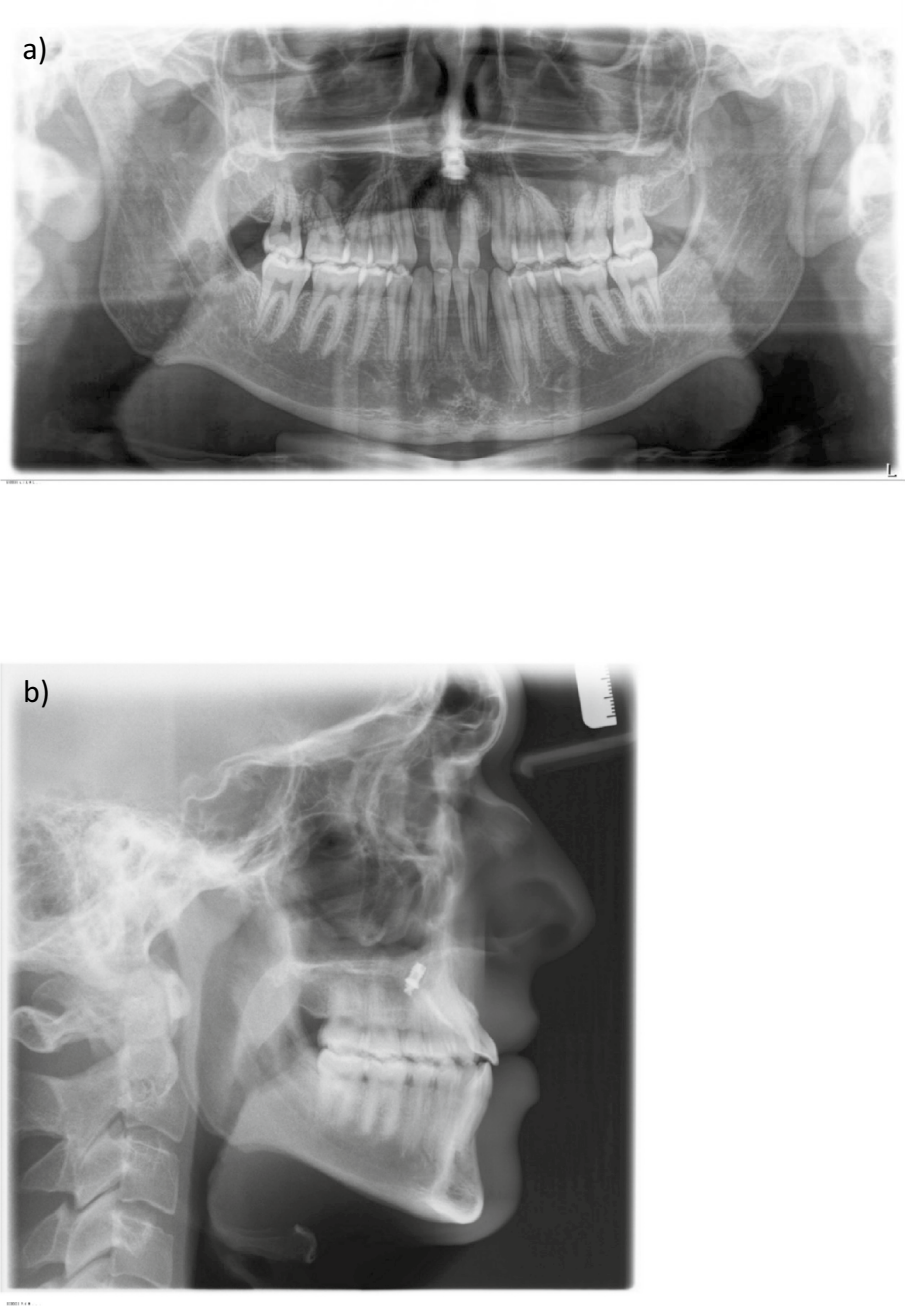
a Panoramic X-ray at the end of treatment b Lateral cephalometric image at the end of treatment
